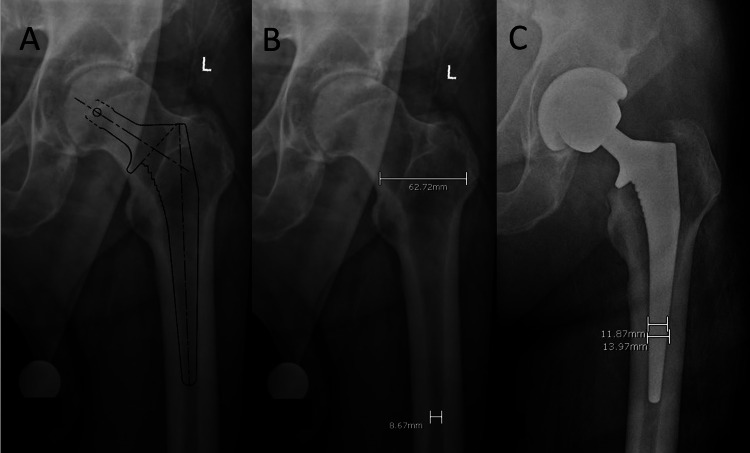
Radiographic examples of templating and measurements. (A) Calibrated template for stem size, hip length, and offset. (B) Sample measurements used to calculate Noble canal-flare index. (C) Sample measurements used to calculate stem-to-canal fill percentage.
